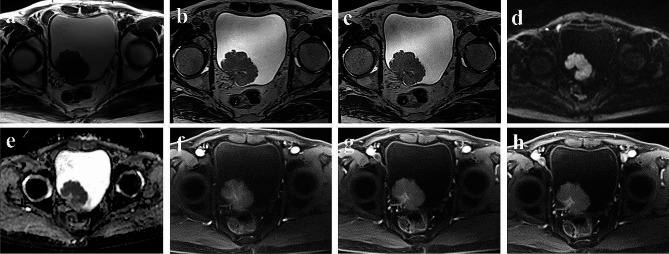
(a-h) display a bladder tumor located at the right ureteral orifice, pathologically diagnosed as non-muscle invasion. Axial T2WI (a), T2-weighted-SPACE (b), CS-T2-weighted-SPACE (c), DWI (d), ADC (e), arterial phase (f), intravenous phase (g), and delayed phase (h)
